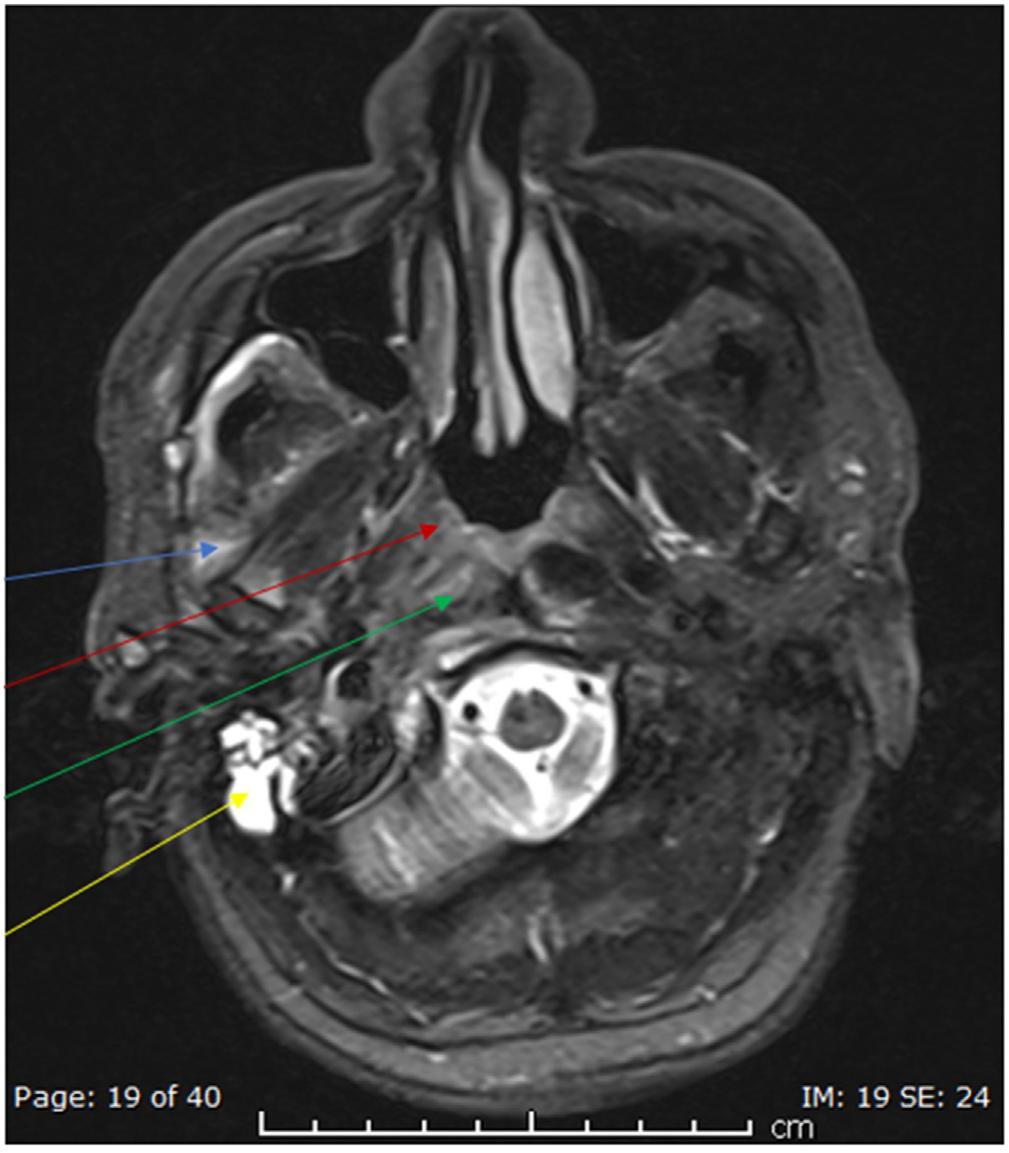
Axial STIR MRI sequence demonstrates hyperintensity (oedema) in multiple neck spaces including masticator space adjacent to the lateral pterygoid muscle (blue arrow). There is mucosal congestion within the nasopharynx (red arrow) with high signal noted posteriorly in the longus capitis muscle (green arrow) suggestive of extension through the retropharyngeal space. Note fluid secretions within the right mastoid air cells (yellow arrow). (For interpretation of the references to colour in this Figure legend, the reader is referred to the web version of this article).
